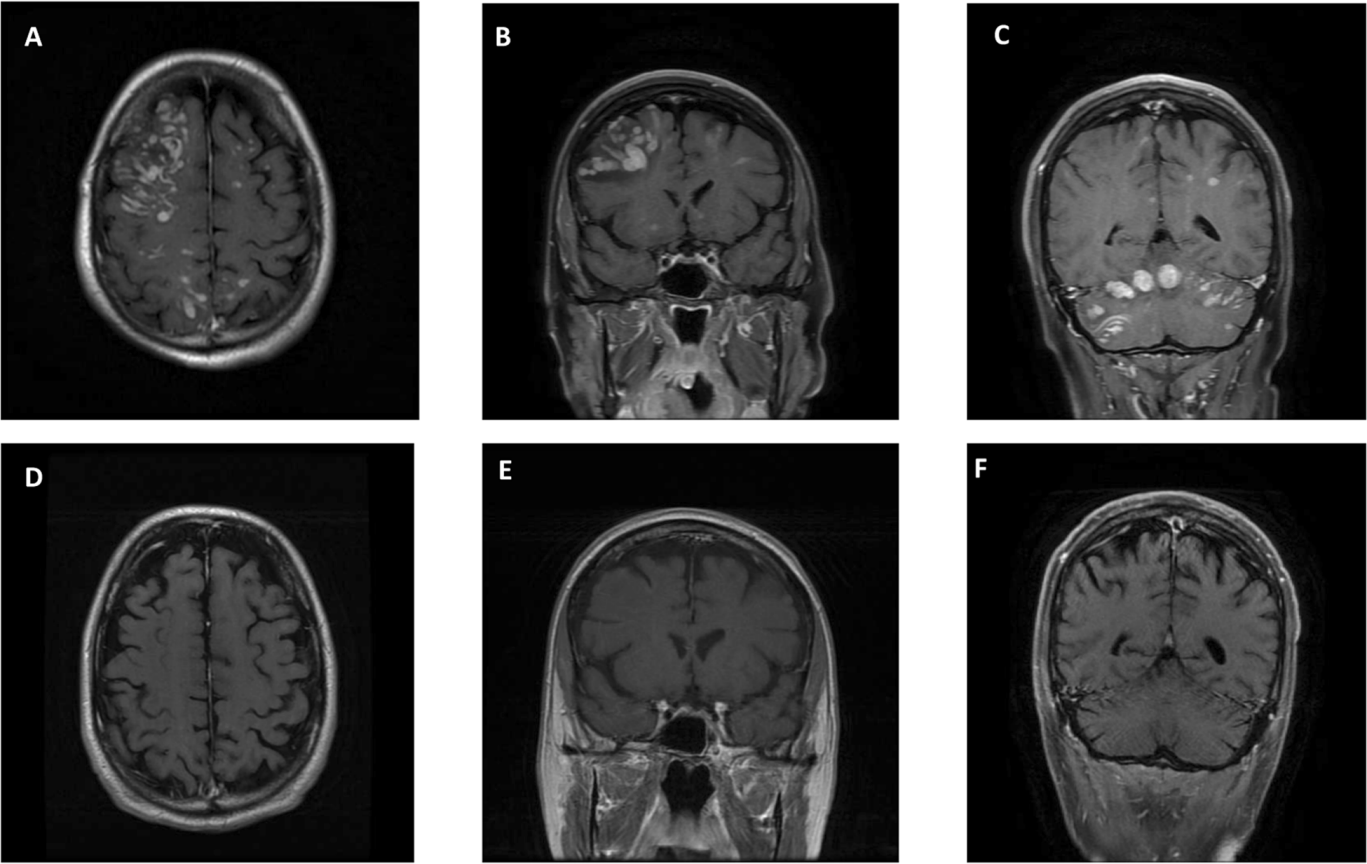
MRI Brain before and after Loralatinib treatment. A, B, C MRI of the brain before starting Lorlatinib (pre-treatment) showing innumerable diffuse enhancing lesions with surrounding vasogenic edema, with a right frontal lobe and bilateral cerebellar predominance. D, E, F Treatment response to lorlatinib with markedly decrease in size and number of brain metastasis and markedly improved cerebral edema.
